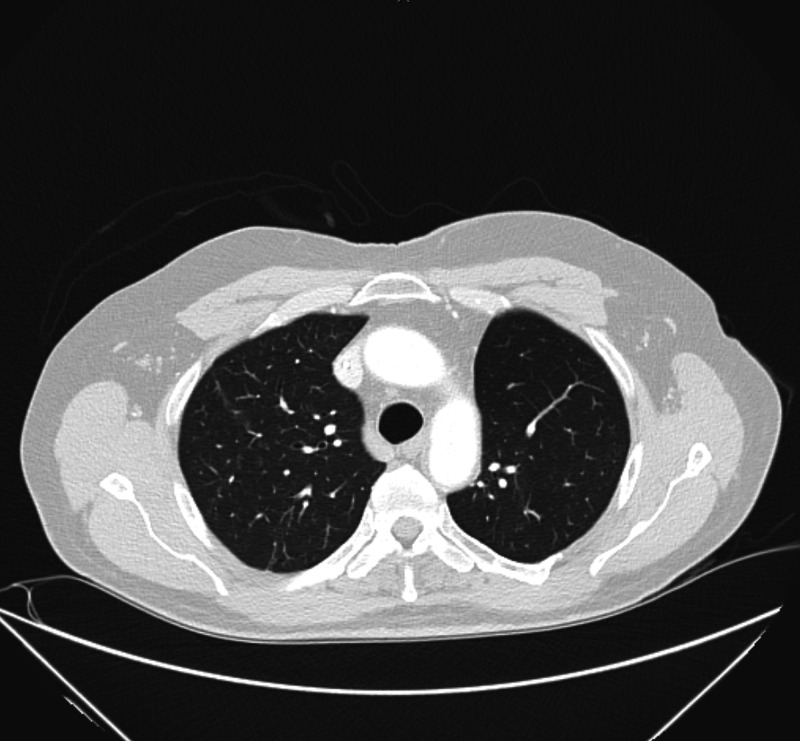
CT imaging of the chest after four years of erlotinib treatment showing no mass or metastases involving the lung CT: computed tomography
